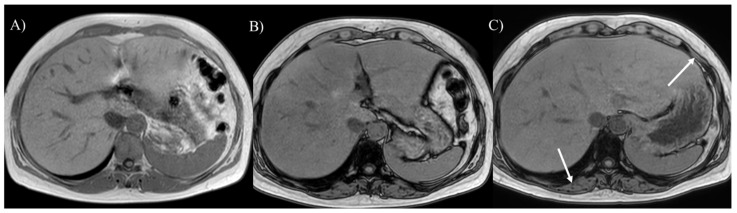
A 16-year-old boy with glycogen storage disease type Ib. Compared to in-phase T1-weighted imaging (A), opposed-phase T1-weighted imaging (B) reveals a signal drop in the liver, suggesting hepatic steatosis. After 7 years, the liver signal almost normalizes, whereas the spinal erector and intercostal muscles do not grow fully (C), (arrows).
